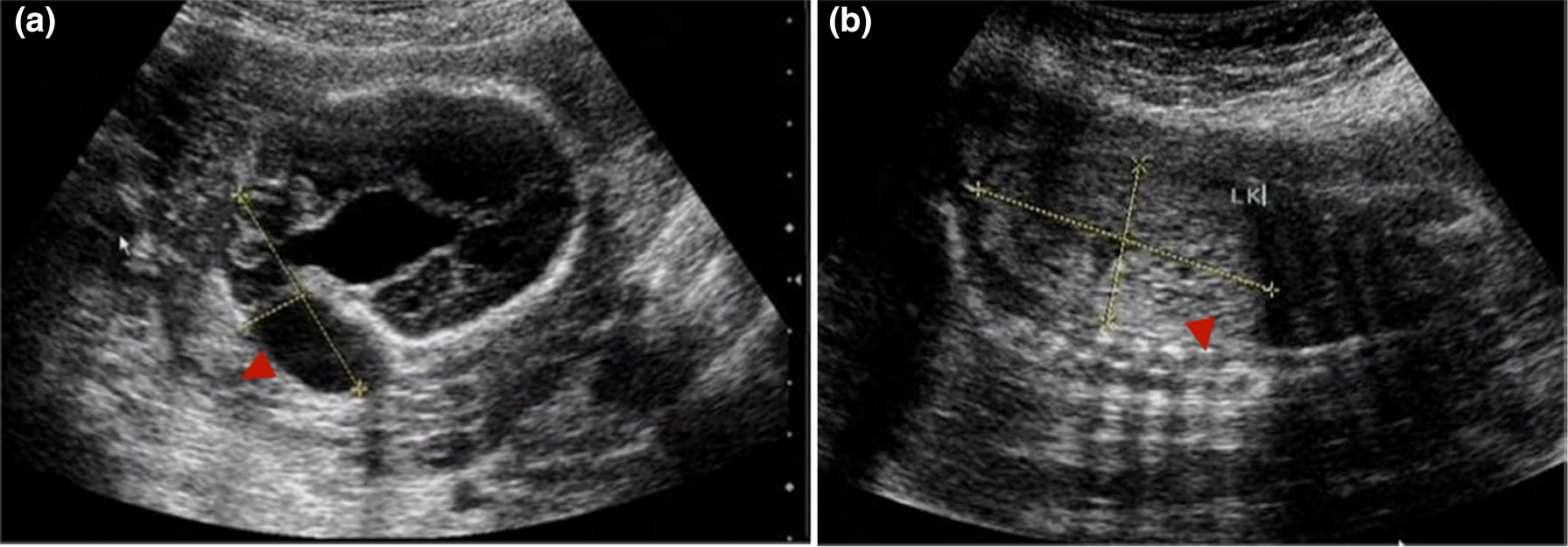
(a) Ultrasound image of fetal meningoencephalocele. The meningoencephalocele was approximately 4.4 cm × 1.3 cm in size (red arrowhead indicates meningoencephalocele). (b) Ultrasound image of the left kidney of the fetus. The left kidney was approximately 5.1 cm × 2.7 cm in size (red arrowhead indicates left kidney).
